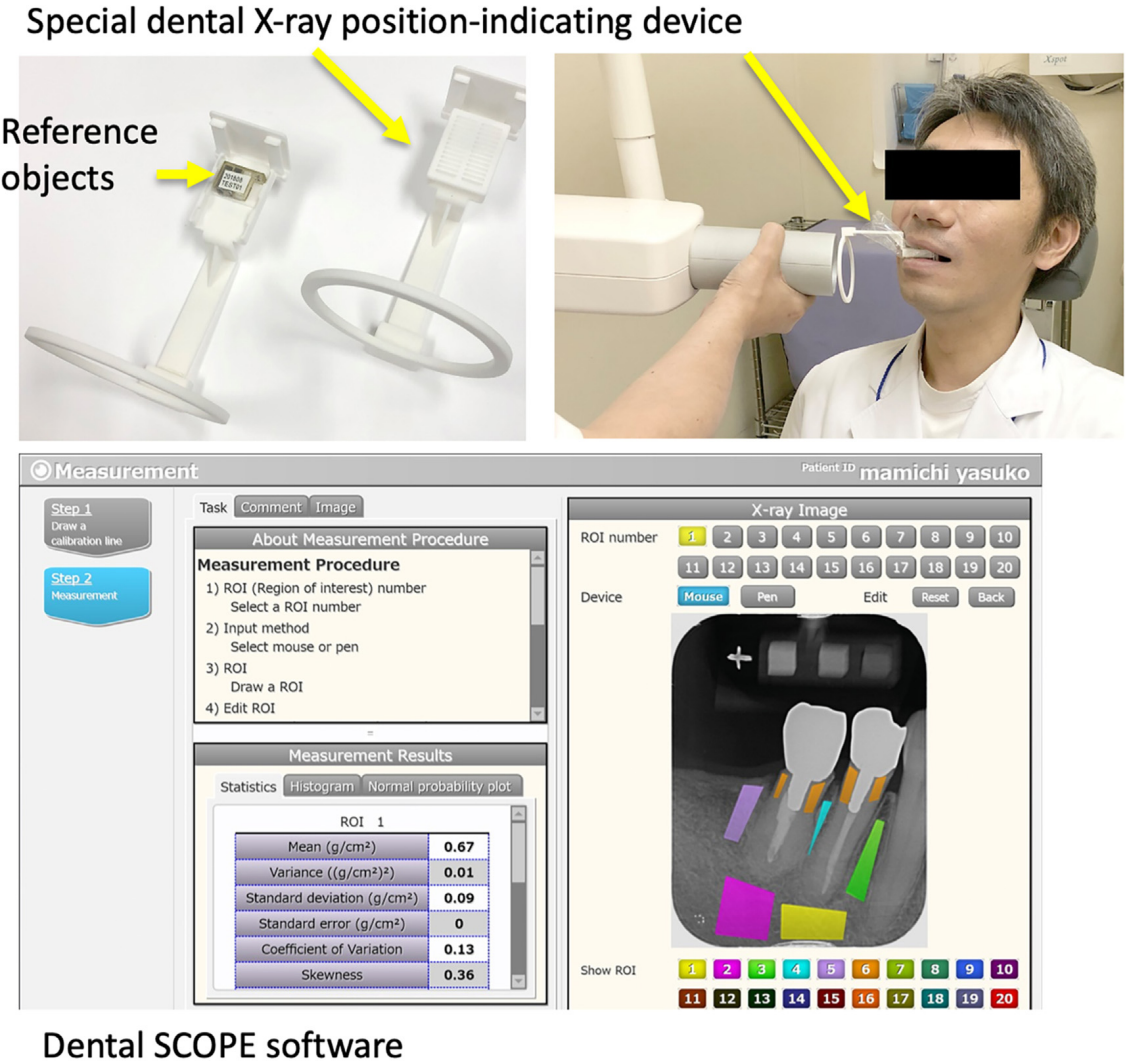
Dental SCOPE system. The BMD measurement system consists of a reference object, an X-ray indicating device, and software.
